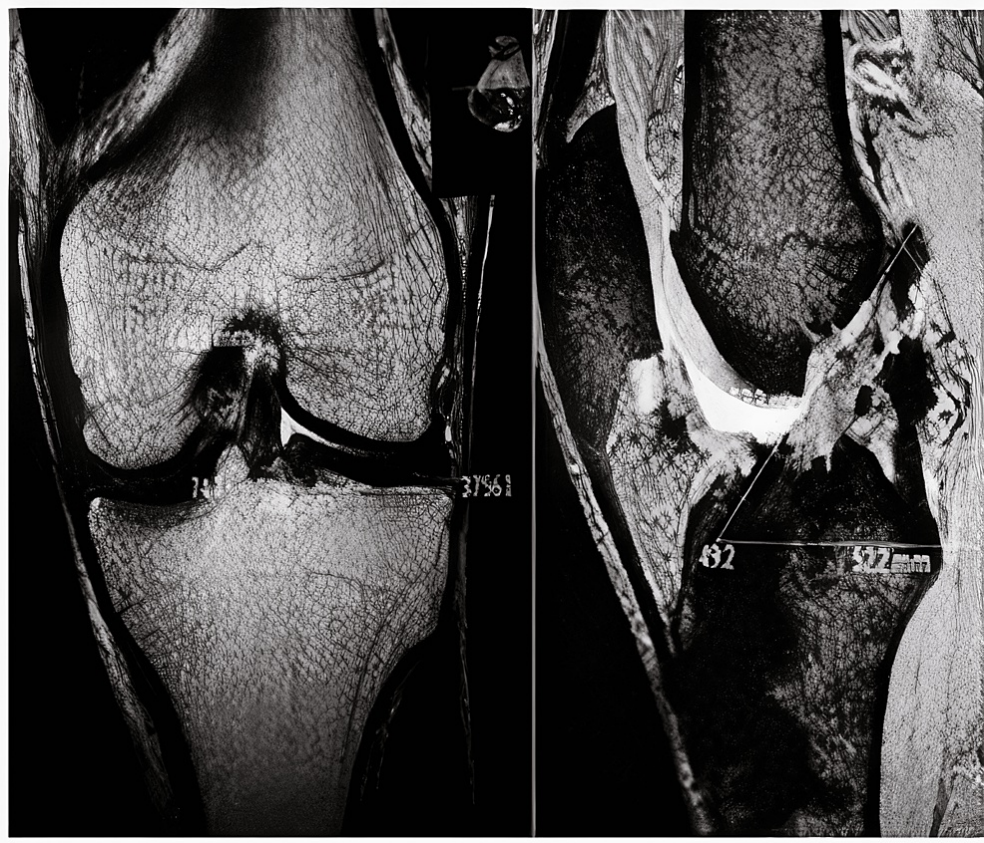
MRI showing the knee with single-bundle reconstruction
